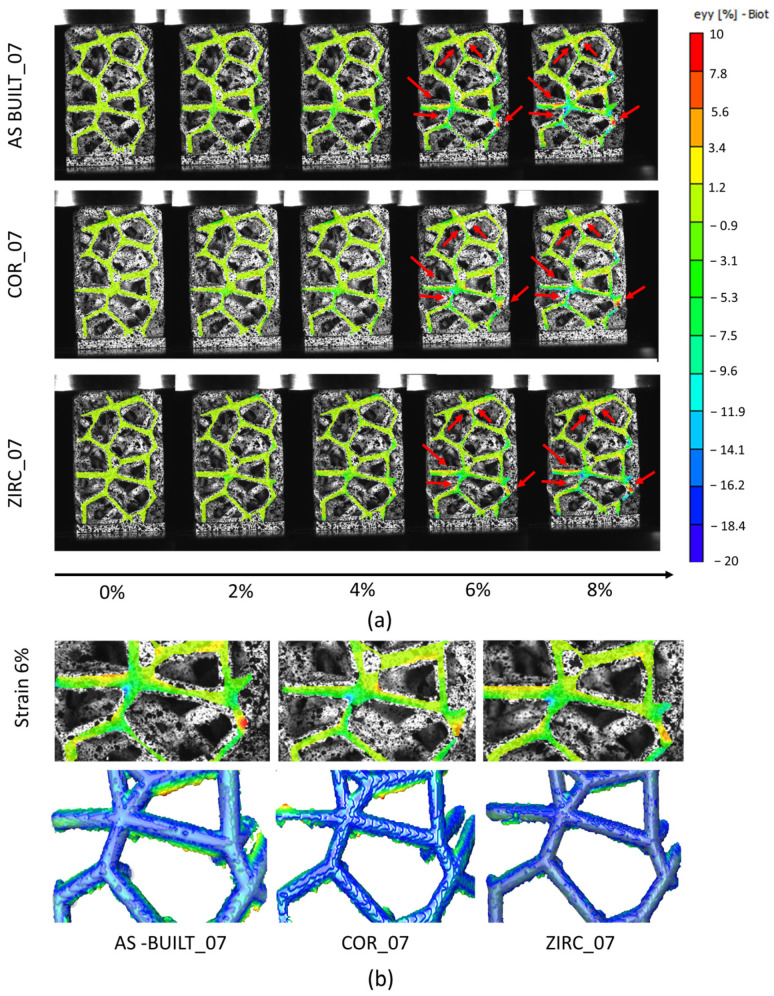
DIC results for sample_07 at different strain values. Red arrows indicate the points with higher local strain values (a); comparison of DIC results at 6% of strain and dimensional deviation from μ-CT (b).
